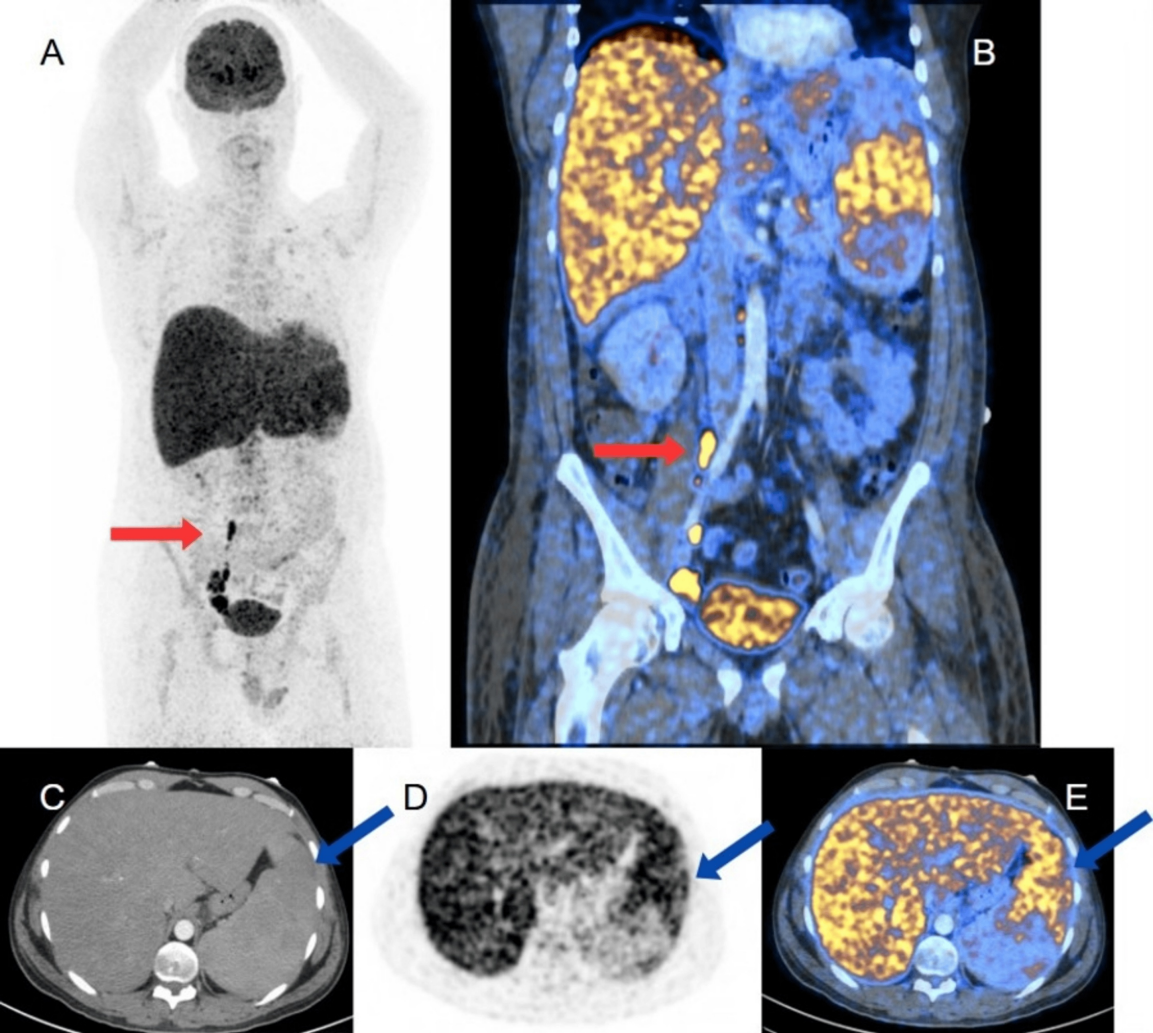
18F-FDG PET-CT images (A) Coronal PET and (B) Coronal PET/CT fusion show increased focal metabolic activity in the spleen and abdominopelvic lymph nodes (red arrows), and diffuse metabolic activity in the liver, associated with hepatosplenomegaly. (C) Axial CT in arterial phase, (D) Axial PET, and (E) Axial Lumbar Interbody Fusion demonstrate a heterogeneous spleen with multiple lesions (blue arrow) and a liver with diffuse metabolic increase. 18F-FDG: 2-deoxy-2-[^fluorine-18^]fluoro-D-glucose
